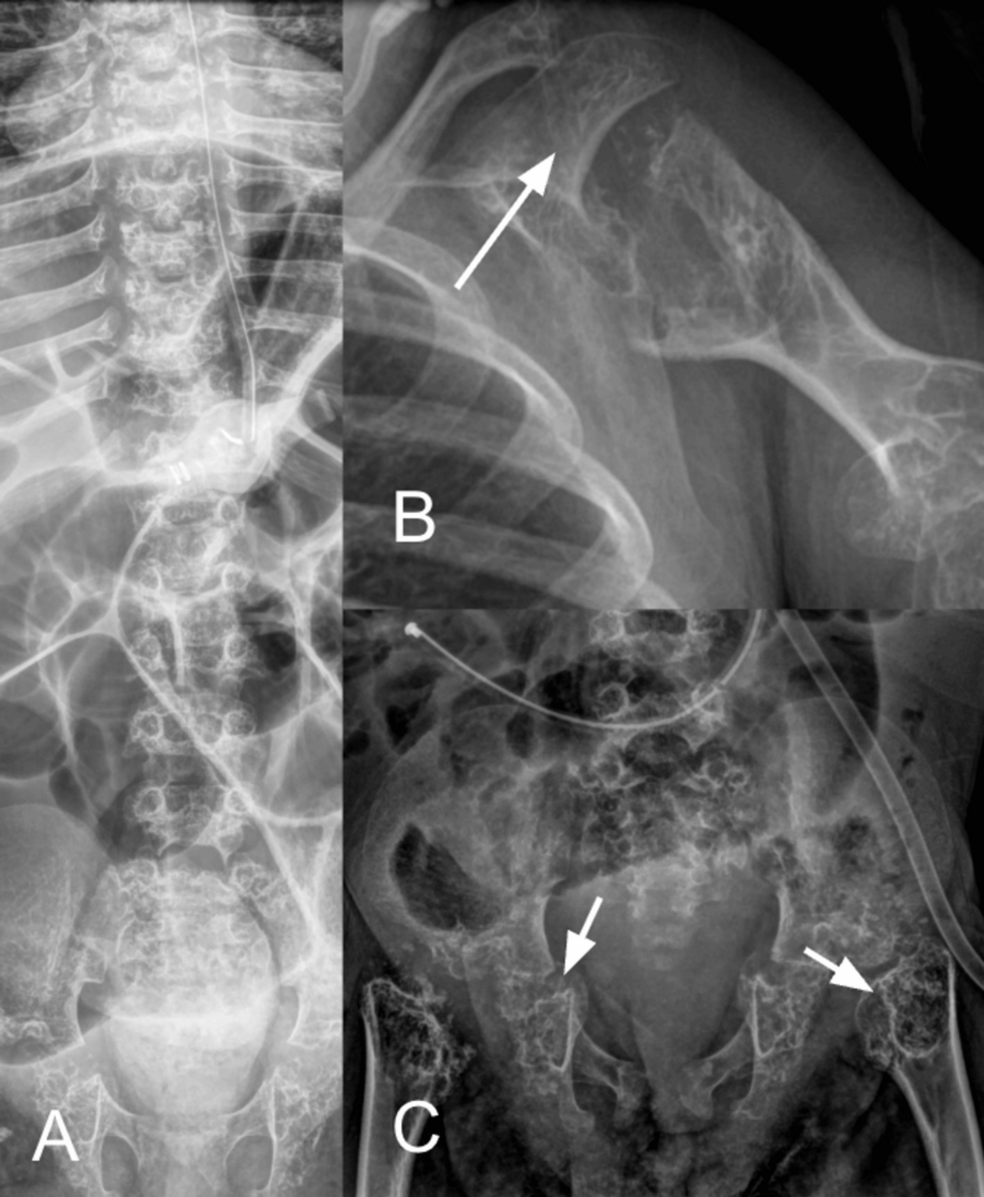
X-rays of the spine (A), left humerus (B), and pelvis (C) Characteristic magnified radiographs of the spine, left humerus, and pelvis show diffuse punctate cartilaginous calcifications involving all osseous structures (A–C). Multiple sclerotic lesions are noted at the epiphyses, associated with generalized rhizomelic shortening of the long bones. There is epiphyseal and metaphyseal widening, more evident in the proximal humerus (B, white arrow) and pelvis (C, white arrows). The vertebral bodies demonstrate punctate calcifications with preservation of height and alignment. Pelvic radiograph (C) further highlights irregular acetabular development with bilateral shallow acetabular roofs. No acute fractures or dislocations are identified.
